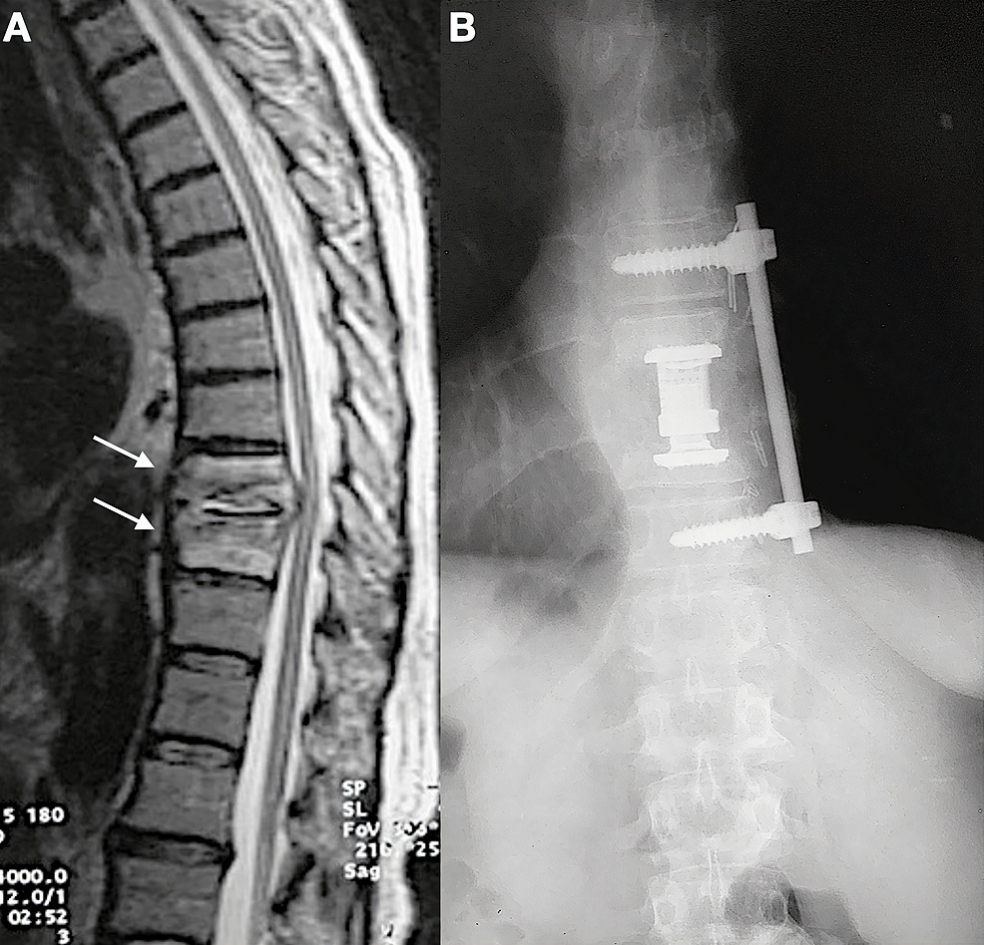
A. Preoperative sagittal T2-weighted image of the lumbar and thoracic spine, showing T10-T11 spondylodiskitis (arrows); B. Postoperative anteroposterior radiograph after the introduction of a titanium mesh cage and anterior instrumentation
